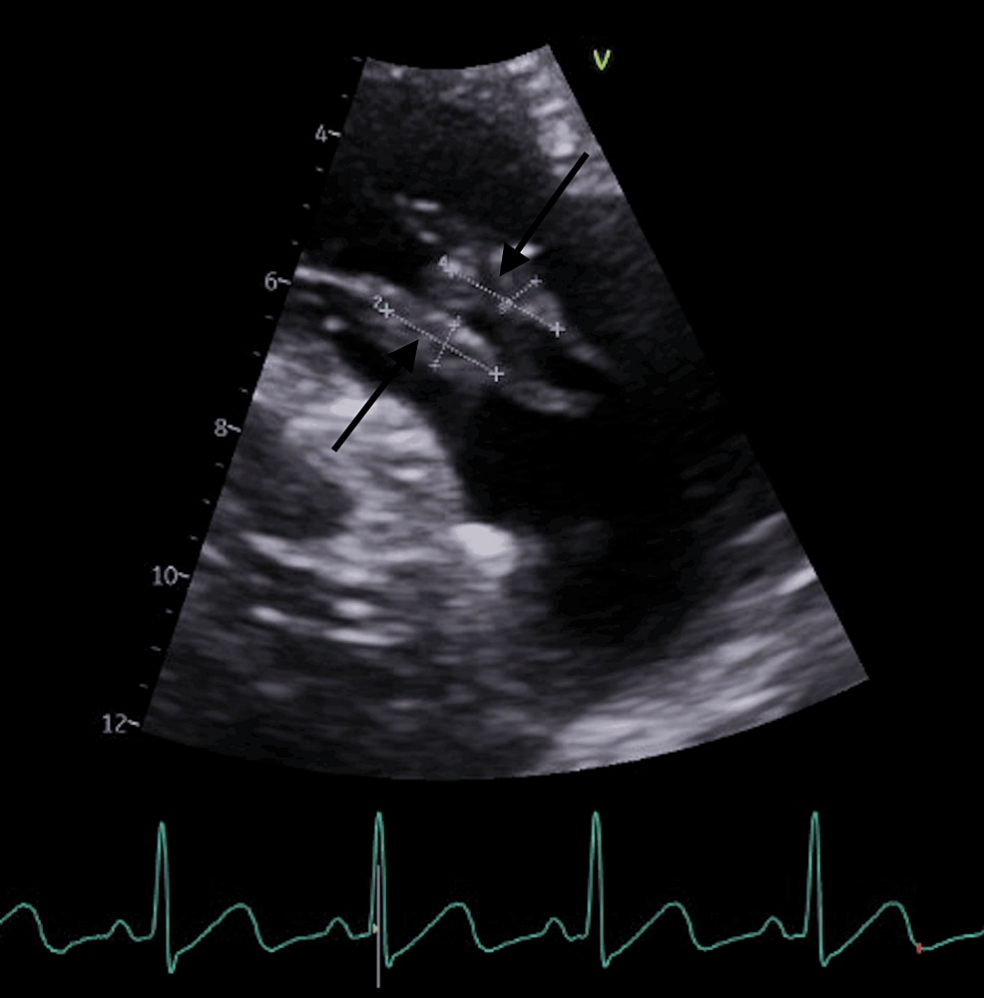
Echocardiogram demonstrating two large vegetations attached to the tricuspid valve
